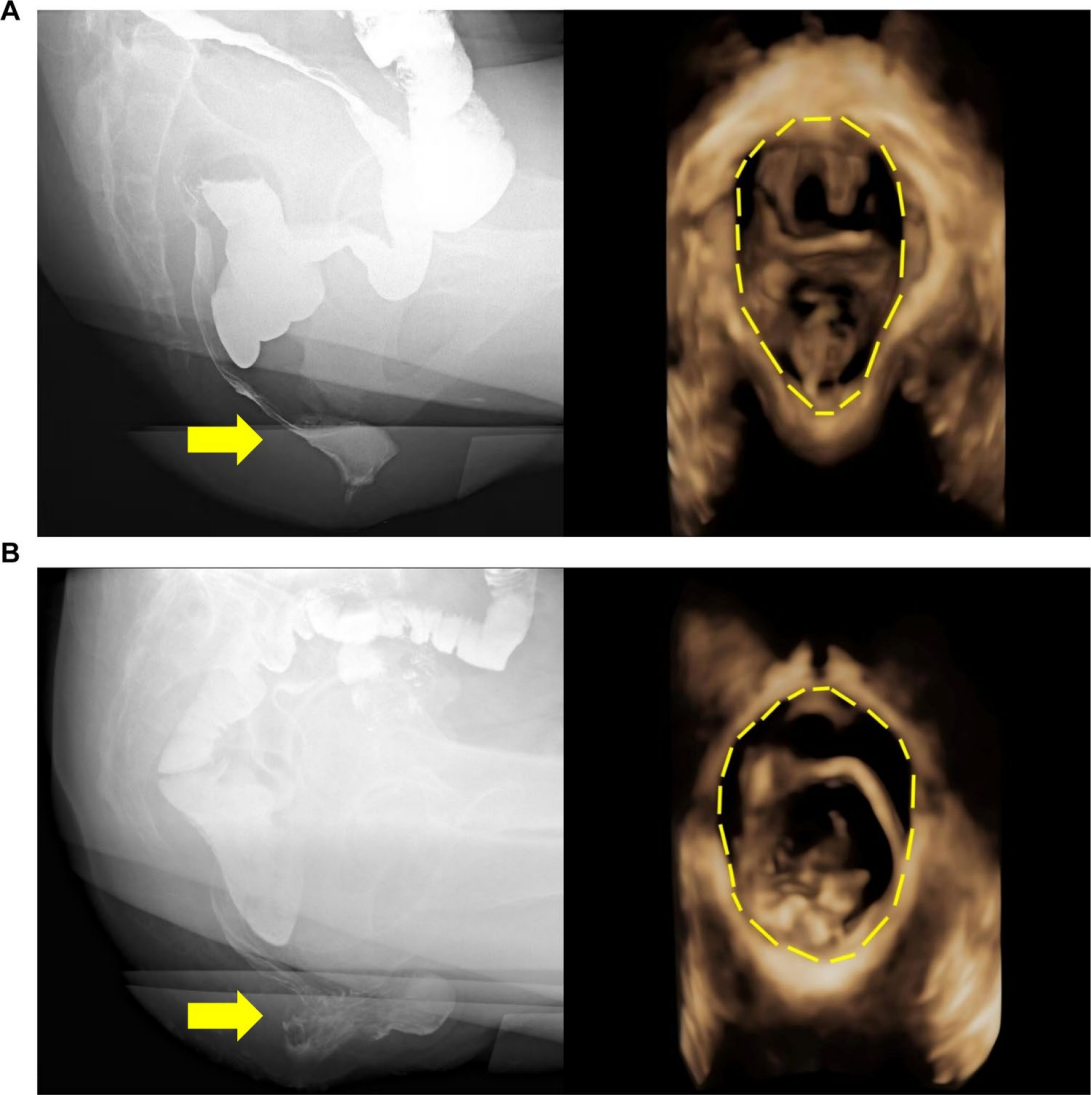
X-ray Defecography and dynamic three-dimensional transperineal ultrasound (3D-TPUS) Images of Mild and Severe Groups. X-ray defecography (recorded at 30 frames/s, 70 kV, 2.5 mA) and dynamic 3D-TPUS (penetration depth 6.1 cm, frame rate 5 Hz) of (A) the mild group and (B) the severe group
